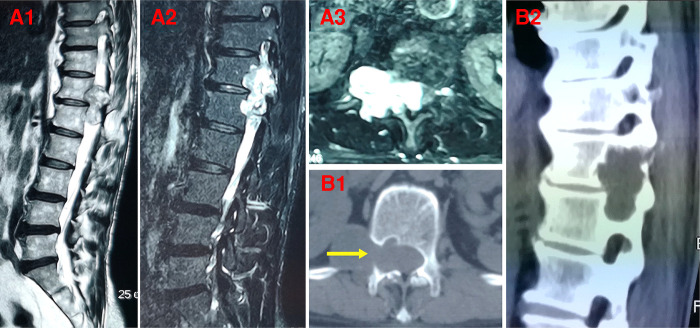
Preoperative MRI and CT showed thoracic dumbbell-shaped tumor of a 58-year-old male patient (case 15) (A,B). (A1, A2) sagittal MRI; (A3) axial MRI; (B1) axial CT; (B2) sagittal CT. The pedicles of adjacent vertebra to thoracic dumbbell-shaped tumor (T12/L1) were involved and damaged. The yellow arrow indicates the damaged pedicle.
